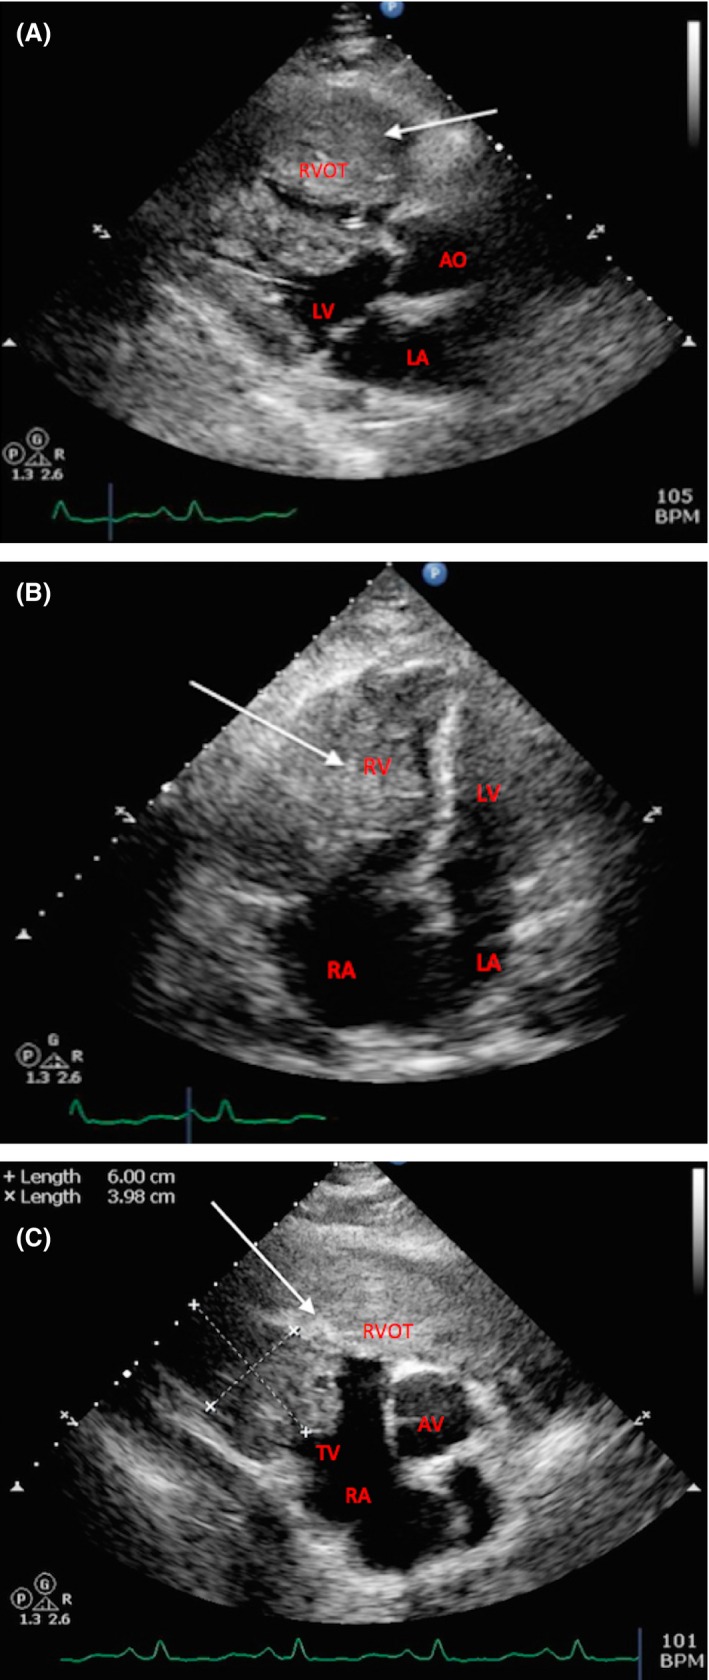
Transthoracic echo of patient 11. (A) Parasternal long‐axis view showing a small LV with normal function and a large 7.7 × 5.7 × 3.5 cm mass (arrow) causing an almost complete obstruction of RVOT, narrow pulse pressure, and low cardiac output; (B) apical 4 chamber view showing an obstructive RV mass (arrow) involving both septal and lateral walls of the LV and extending from base to apex; (C) short‐axis view at the level of AV showing RVOT mass (arrow) extending from the level of TV toward the pulmonic valve (RVOT, right ventricular outflow tract; AO, aorta; LV, left ventricle; LA, left atrium; RV, right ventricle; RA, right atrium; AV, aortic valve; TV, tricuspid valve).
